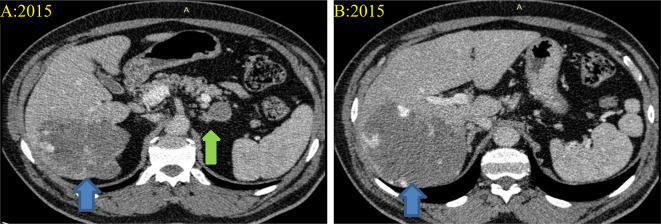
Computed Tomography (CT) scans at the level of the of the liver and adrenal glands (A & B) which reveal a large liver lesion with peripheral nodular enhancement and matching of the blood pool measuring about 5 × 7.5 cm compatible with his known hemangioma (Blue arrows). Left adrenal lesion of about 3 × 3 cm with an average density (0 HU) consistent with adrenal adenoma (Green arrow)
